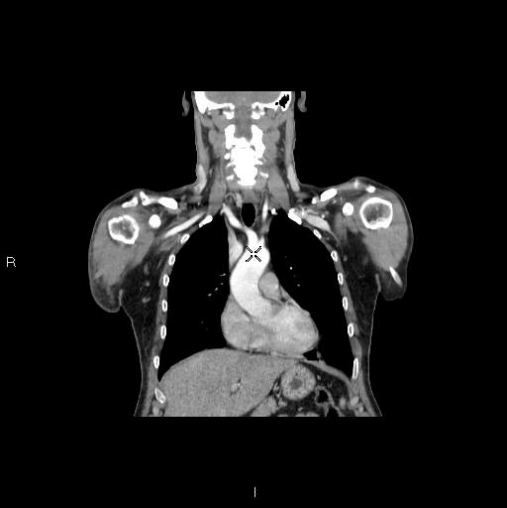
Chest angio-CT shows diffuse thrombosis of the anonyma and subclavian veins and distal tract of the right axillary vein, without involvement of the pulmonary circulation.
